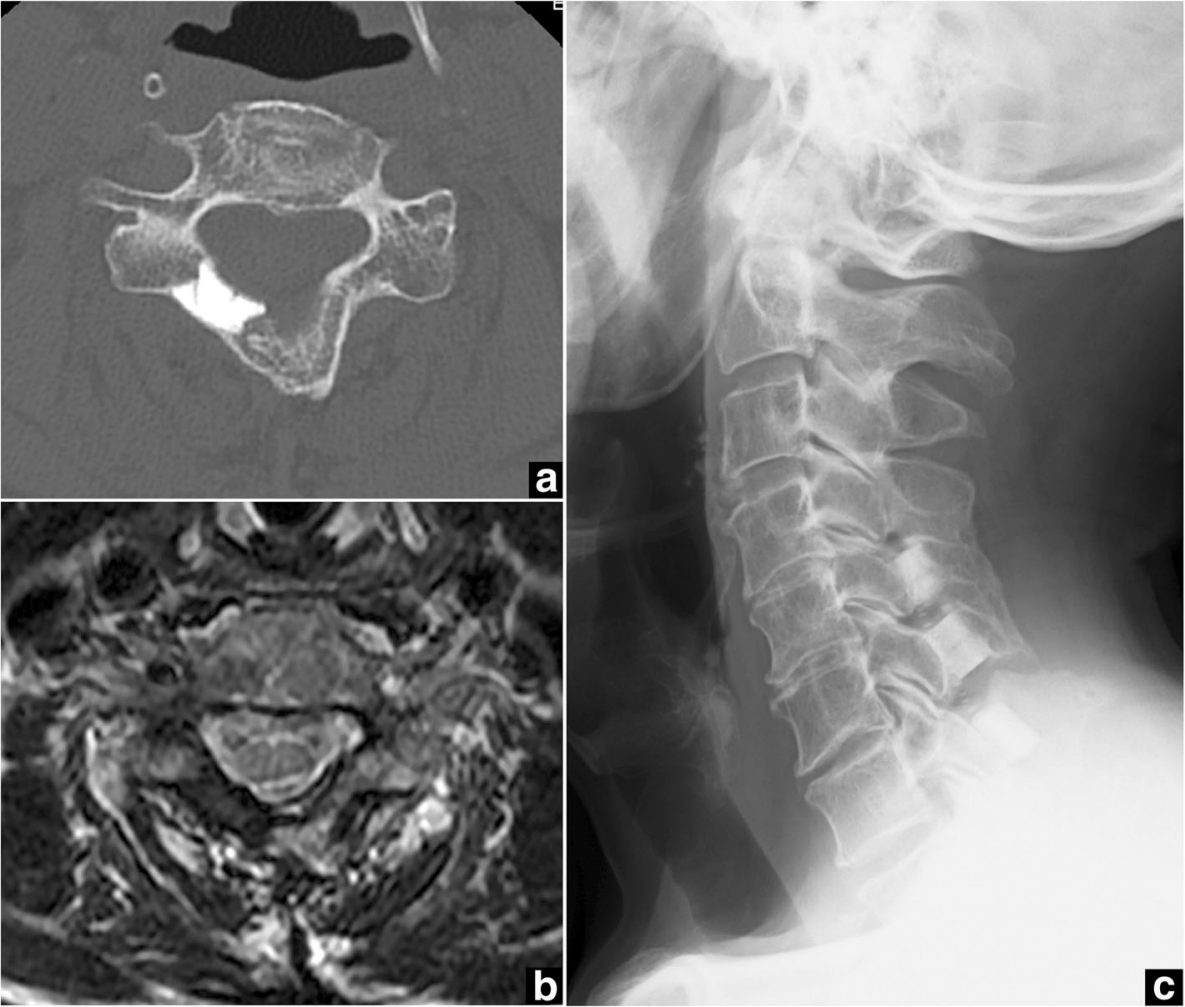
Case 1. Postoperative computed tomography (CT), magnetic resonance imaging (MRI), and plain X-ray of the cervical spine. a Axial CT at the C6 level obtained 5 years after surgery demonstrates a newly created spinal canal with HA spacers bonded to host bone. b Axial MRI at the C6 level obtained 5 years after surgery shows no tumor recurrence with good visualization inside the spinal canal. c Lateral plain X-ray obtained 6 years after surgery shows normal sagittal alignment
